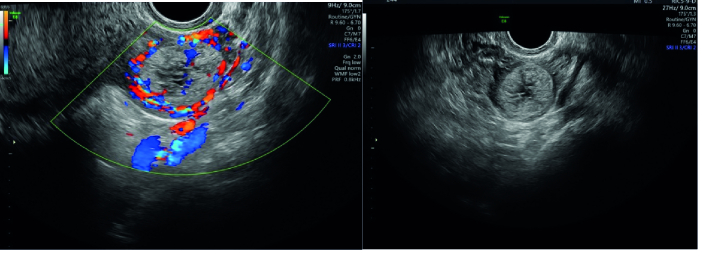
Transvaginal ultrasonography image, molar ectopic pregnancy in left adnexa with snow storm view.
